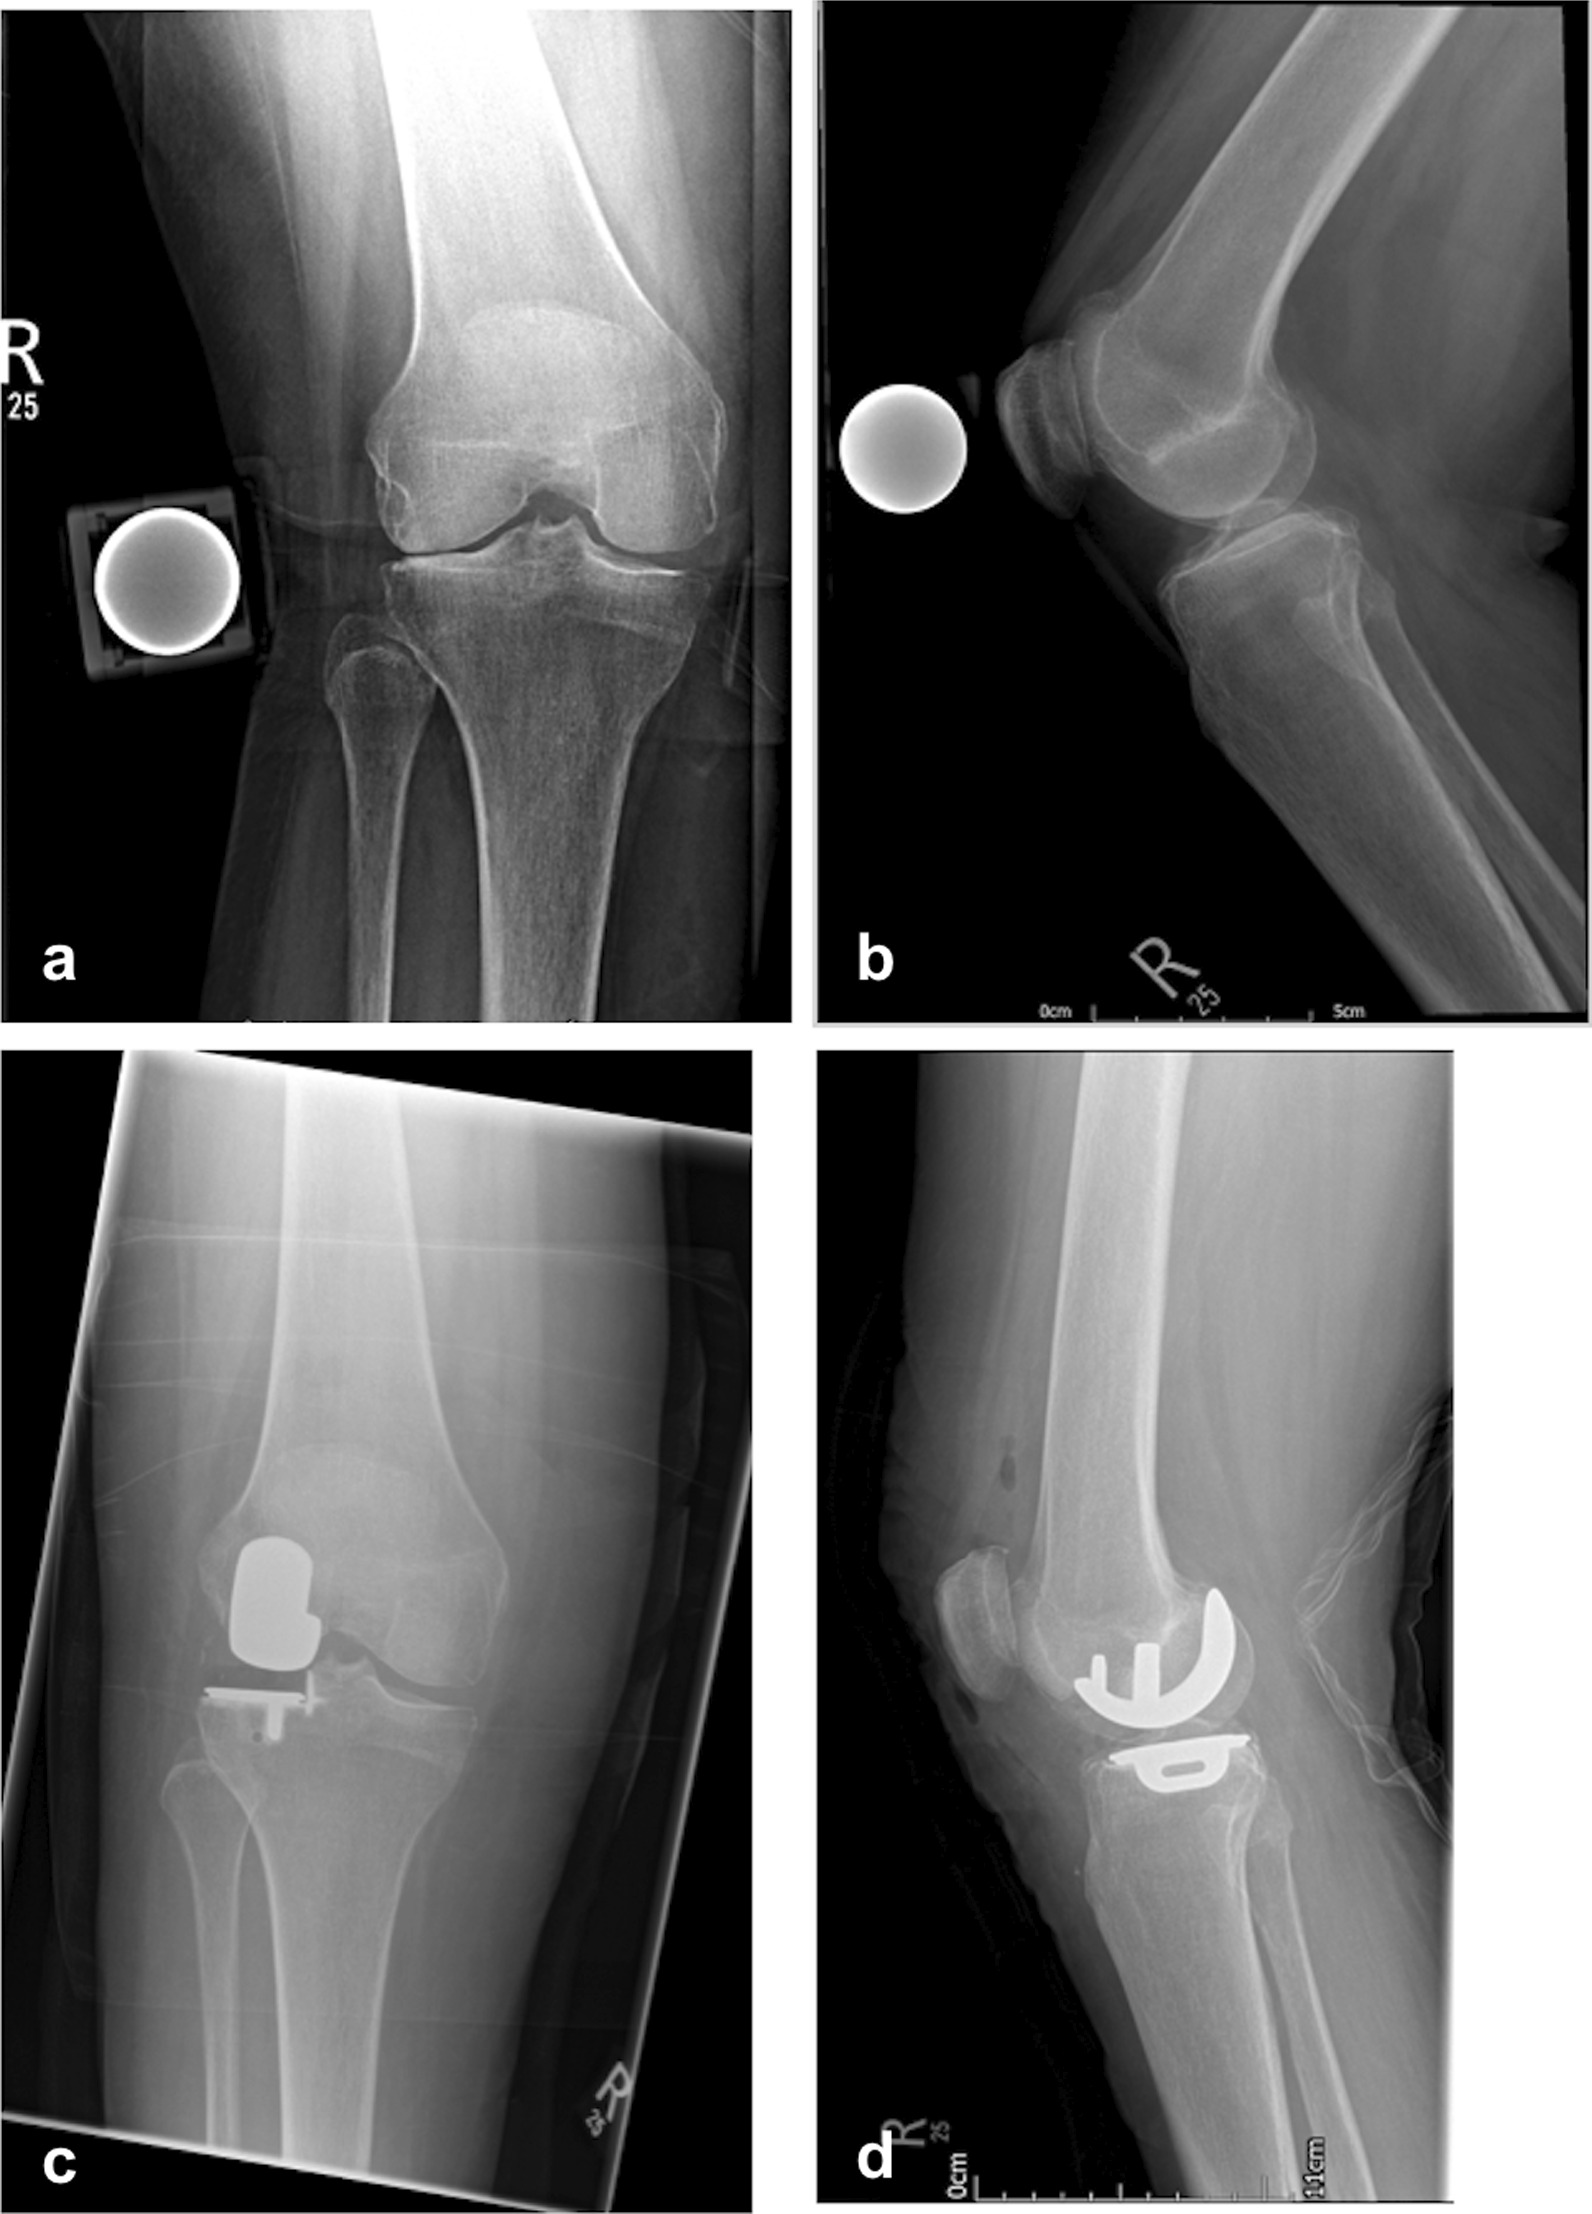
a–d Pre- and postoperative X-ray of the right knee with isolated lateral OA which underwent L UKR
